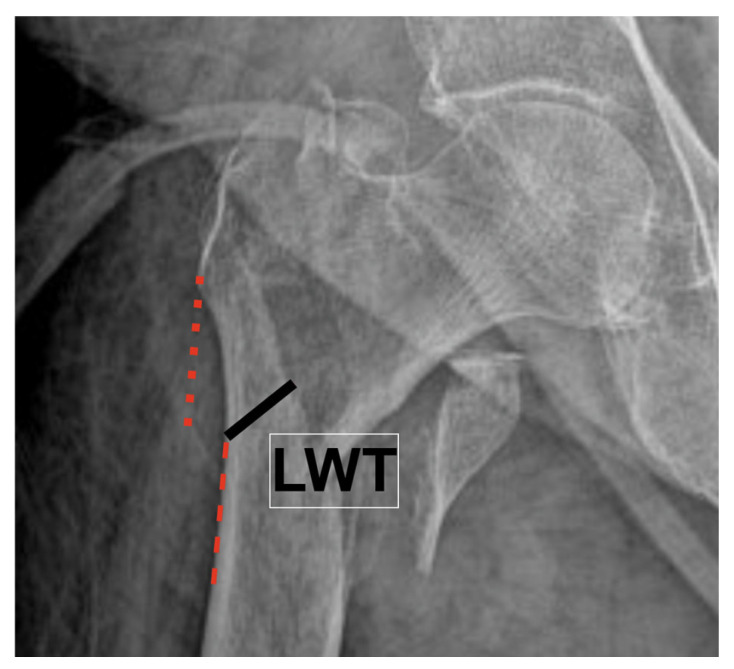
Measurement of lateral wall thickness (LWT) on postoperative anteroposterior (AP) radiograph according to the method described by Palm et al. The thickness of the lateral wall was measured along a line drawn 3 cm below the innominate tubercle of the greater trochanter at an angle of 135° to the femoral shaft axis (black line). The distance between the lateral cortex and the fracture line along this 135° line (red dotted line) represents the LWT.
